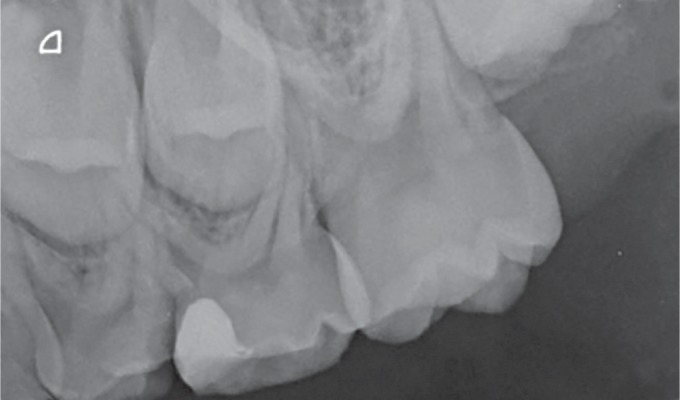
X-ray evaluation of the upper left maxillary primary molar
